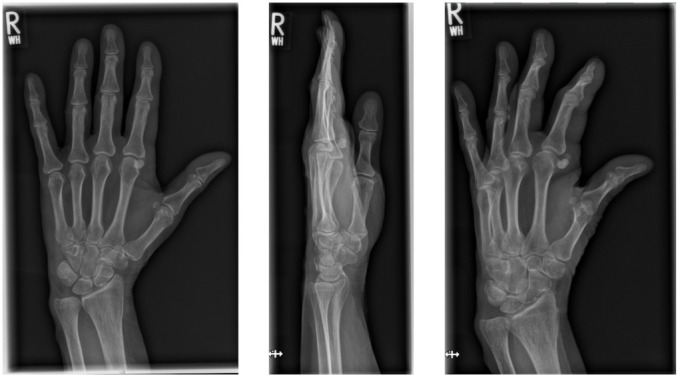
Preoperative X-ray of the right hand in three planes
